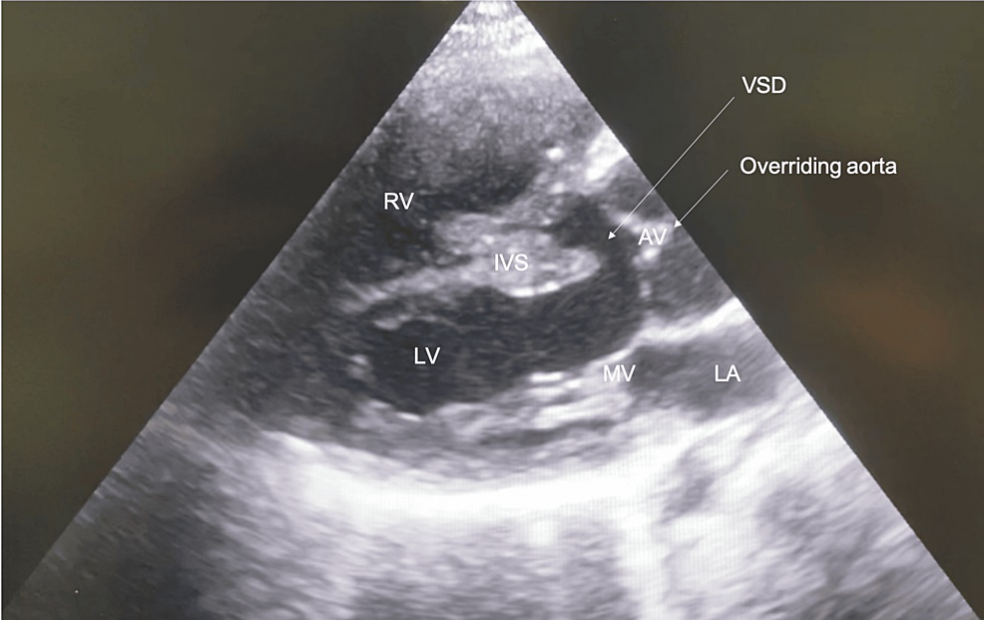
Trans-thoracic echo - parasternal long-axis view LA: Left atrium; MV: mitral valve; LV: left ventricle; RV: right ventricle; IVS: intraventricular septum; AV: aortic valve; VSD: ventricular septal defect
